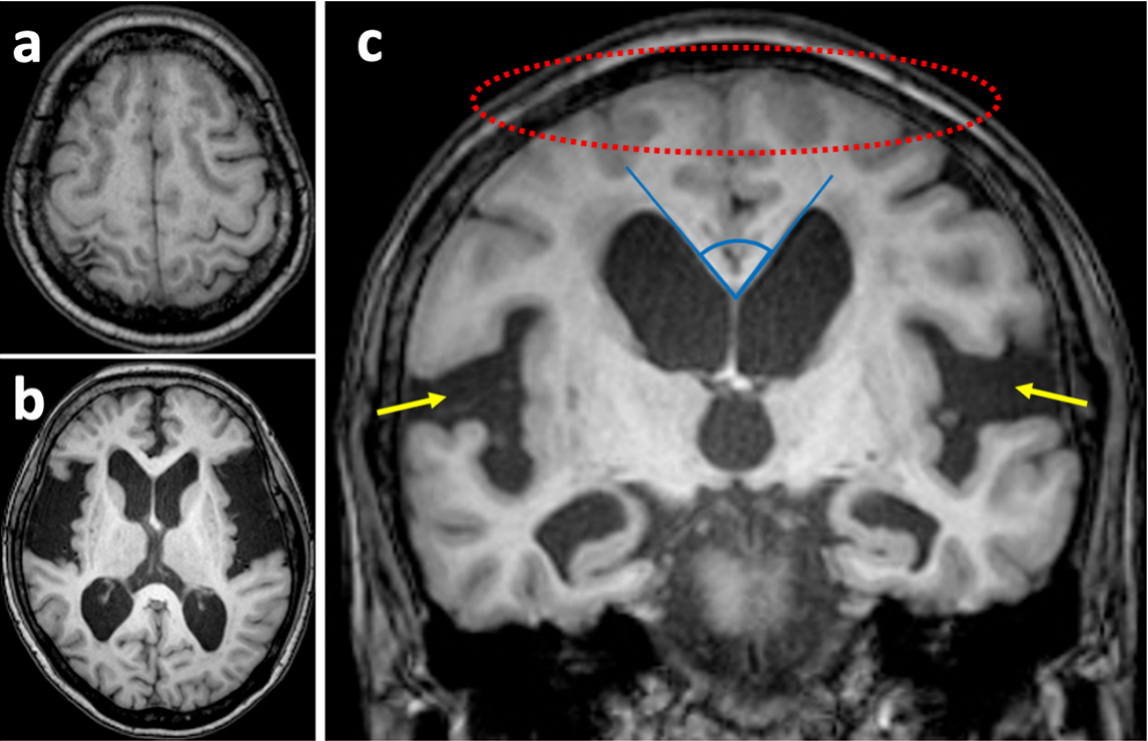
Representative magnetic resonance imaging (MRI) of disproportionately enlarged subarachnoid space hydrocephalus (DESH). Ventriculomegaly, (A) tight sulci in the midline and the high convexity and (B) dilated Sylvian fissures are demonstrated. (C) The callosal angle is the angle between the lateral ventricles measured on the coronal plane, which is perpendicular to the anterior-posterior commissure plane through the posterior commissure of the patients.
